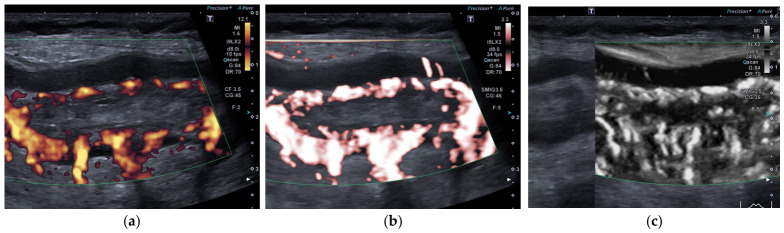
Comparison of power Doppler (a), color-coded SMI (b) and monochrome mode SMI (c) within the same patient; the Limberg classification of power Doppler was IV. Uncropped images are provided in the Supplementary Material.
